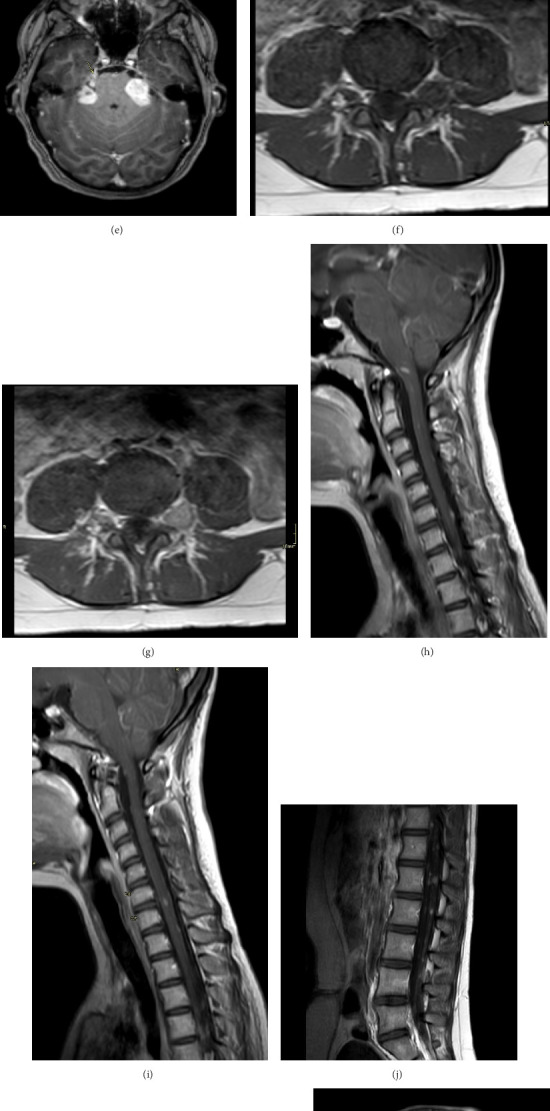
MRI images revealing multiple schwannomas. (a–c) Axial FLAIR, axial T2∗, and axial T1W images post gadolinium administration at the level of the cerebellopontine angles: bilateral solid soft tissue masses in the cerebellopontine angles, showing intracanalicular component with widening the porus acusticus (trumpeted IAM sign) and causing mass effect on the lateral aspect of the pons, middle cerebellar peduncles and cerebellum, with narrowing of the fourth ventricle. Both lesions show high FLAIR signal, intense enhancement post contrast with no diffusion restriction (not shown), and contain foci of hemorrhage, consistent with bilateral vestibular schwannomas. (d, e) Axial T1W image post gadolinium administration shows small enhancing schwannomas along the trigeminal nerve, bilaterally (right is shown), inferior to the Meckel's cave, measuring approximately 8 mm. (f, g) Axial T1W images pre and post gadolinium administration at the L4-L5 level show a 1.3 × 1.4 cm enhancing lesion on the left side in keeping with extraforaminal neurofibroma or schwannoma. (h–j) T1W images of the spine post gadolinium administration show multiple enhancing lesions at the level of the craniocervical junction and within the spine, some of which are intramedullary (at C2-C3, C6-C7) and others along the surface of the cord, corresponding to ependymomas and neurofibromas. Note is made of mild cerebellar tonsillar herniation. (k) Axial T1W image post gadolinium administration at the level of L2 showing 6 mm enhancing lesion along the nerve roots. (l) Axial T1W images post gadolinium administration: subcutaneous enhancing lesions at the level of the left frontal and parietal scalp, representing subcutaneous neurofibromas. (m, n) Axial T1W images post gadolinium administration showing a 7 mm extra-axial, broad-based, enhancing lesion arising from the right anterior clinoid process and another 6 mm lesion along the falx anteriorly in keeping with meningiomas.
